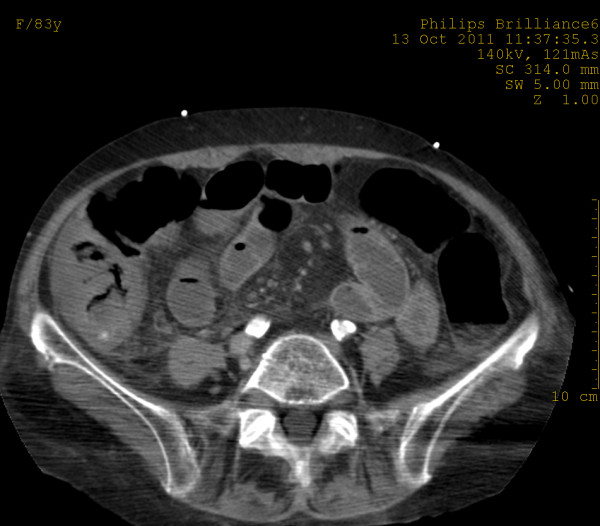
Axial CT image at arterial phase demonstrates a thickened caecal wall. A focal area of high attenuation suggesting active bleeding is seen in the lumen of the caecum.
